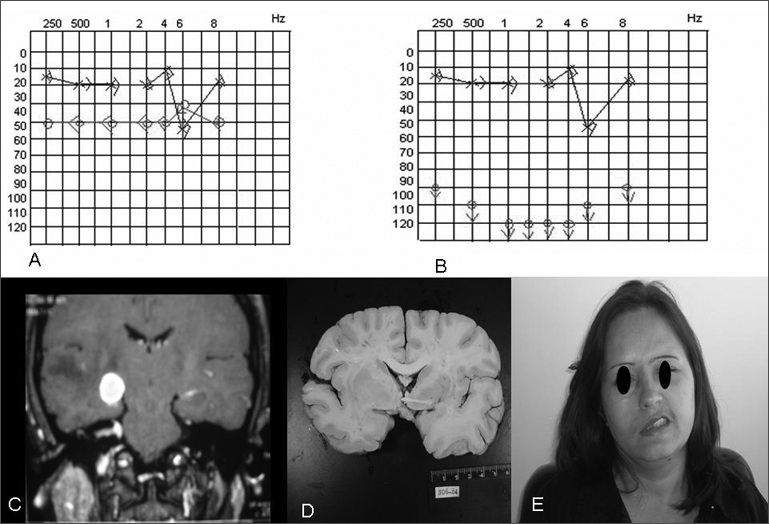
A - initial audio; B - final audio; C - MRI with a metastasis on the MAI; D - necropsy result; E - facial paralysis G V to the left.
